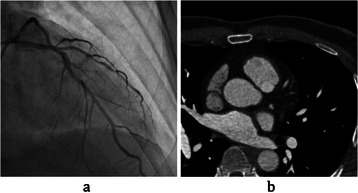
Data for “Patient3”. (a) An example of selected angiogram. (b) An example of a CTA slice.
